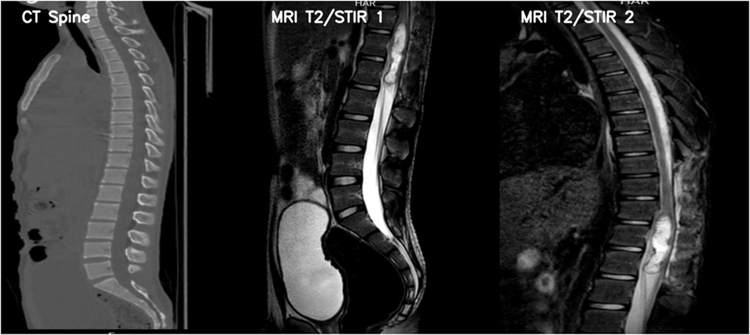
Spinal imaging showing an intradural lesion. (Top) CT scan reveals an increased spinal canal diameter from D11 to L1. (Middle & bottom) MRI T2/STIR sequences demonstrate a well-defined hyperintense lesion, suggestive of a cystic or fatty component, characteristic of an intraspinal teratoma.
